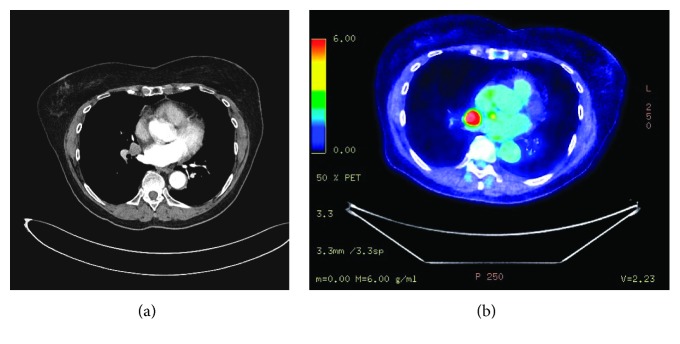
(a) CT scan and (b) PET scan showing PET-positive R12 node.
